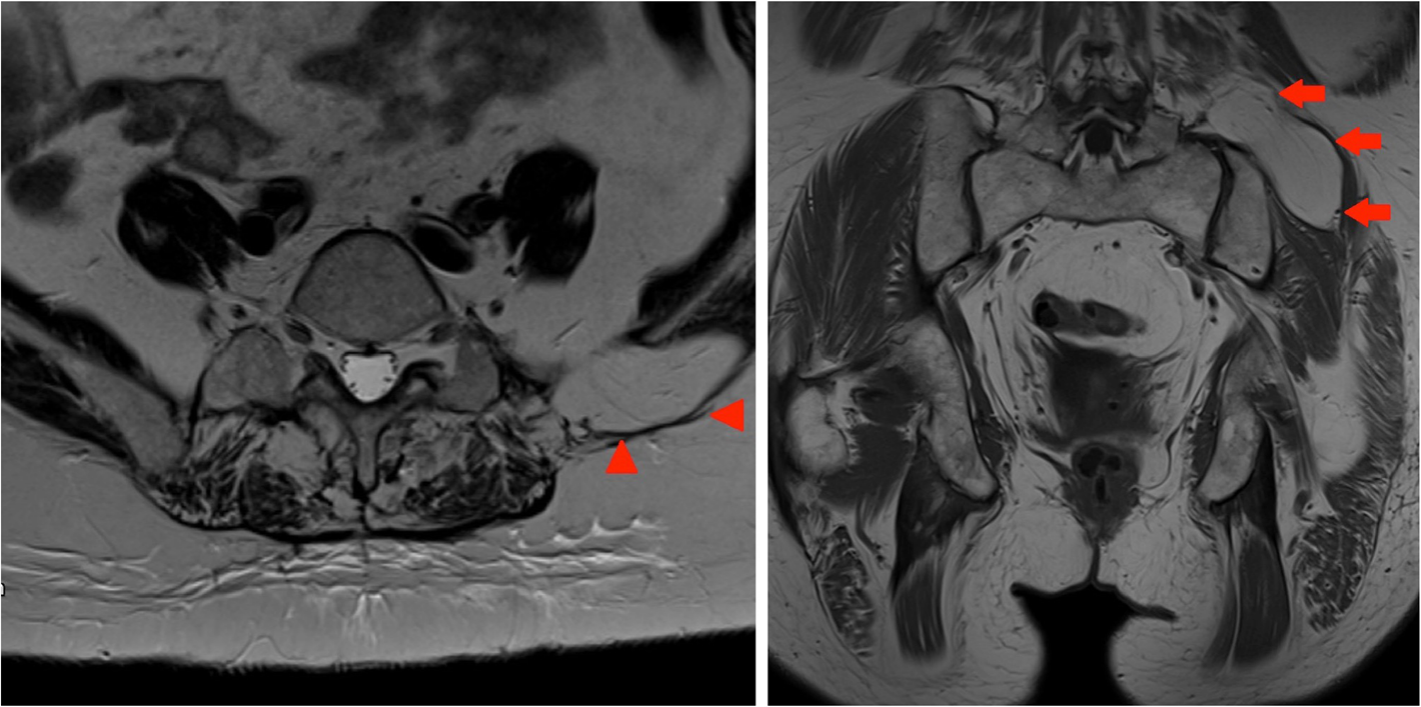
Left panel: Axial T2-weighted image of the pelvis: a fatty mass is seen to extend into the lumbar soft tissues with an intramuscular component in the gluteus medius. Right panel: Coronal T1-weighted image of the pelvis: red arrows outline the lumbar defect with fatty hernia contents
